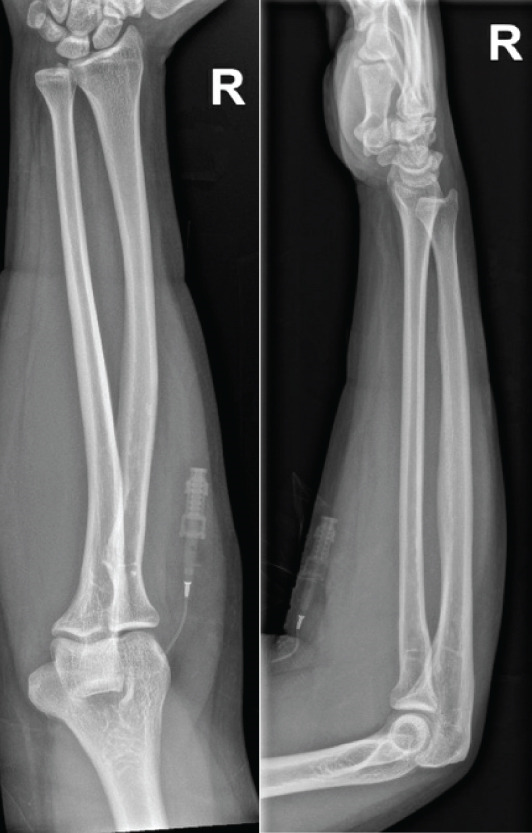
Postoperative radiographs demonstrating anterior radial head dislocation.
